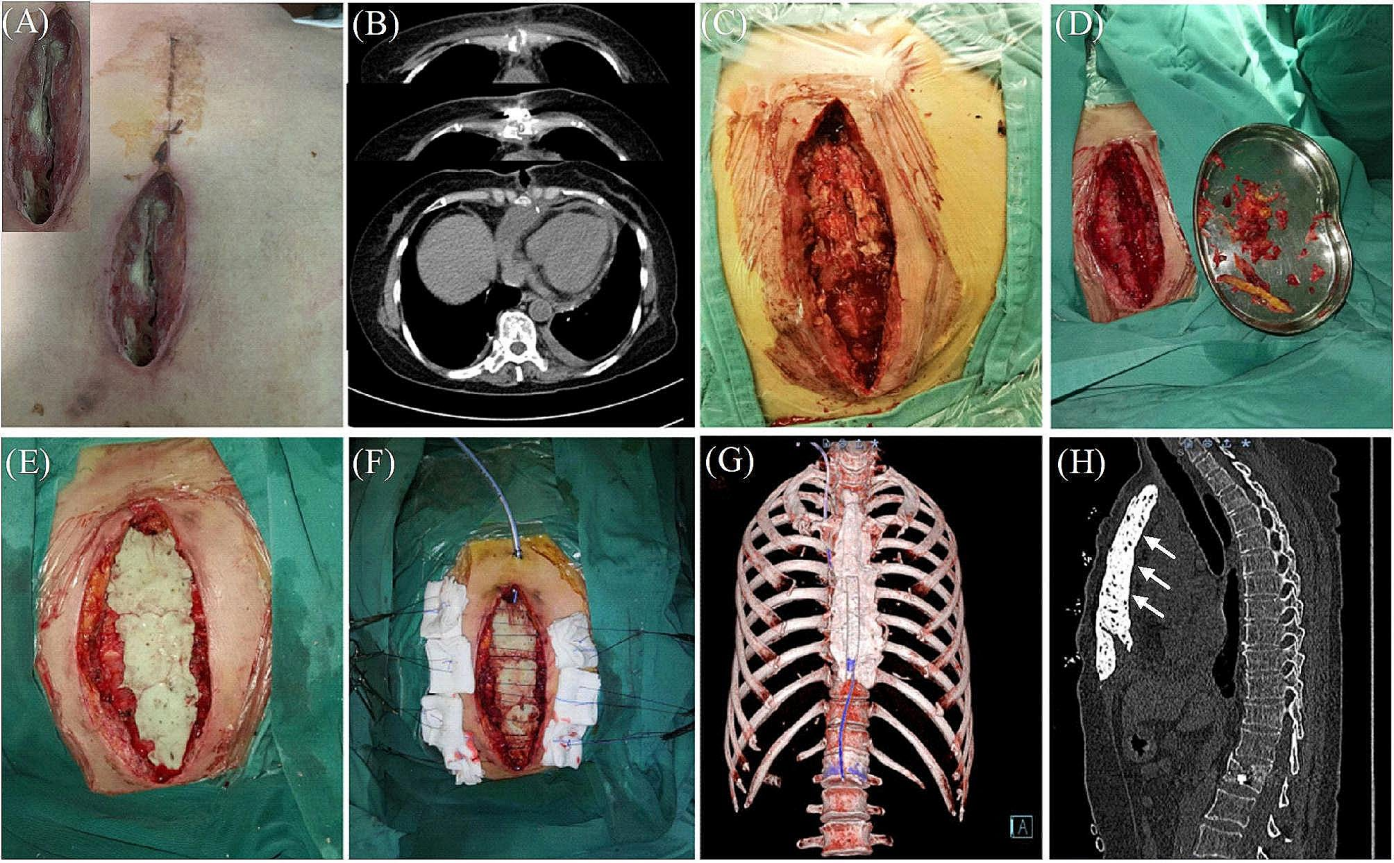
(A) Post-sternotomy sternal dehiscence and necrosis. (B) Preoperative thoracic computed tomography showing sternal dehiscence. (C) Open the wound completely. (D) The wound after debridement. (E) Antibiotic-loaded bone cement was sufficient to cover the whole length of sternum defect. (F) bilateral pectoralis major muscle flaps tension-free suture. (G) Three-dimensional chest computed tomography (CT) scan images showing the results of the chest wall reconstruction using bone cement. (H) The gap between cement and the mediastinal structures (white arrow)
